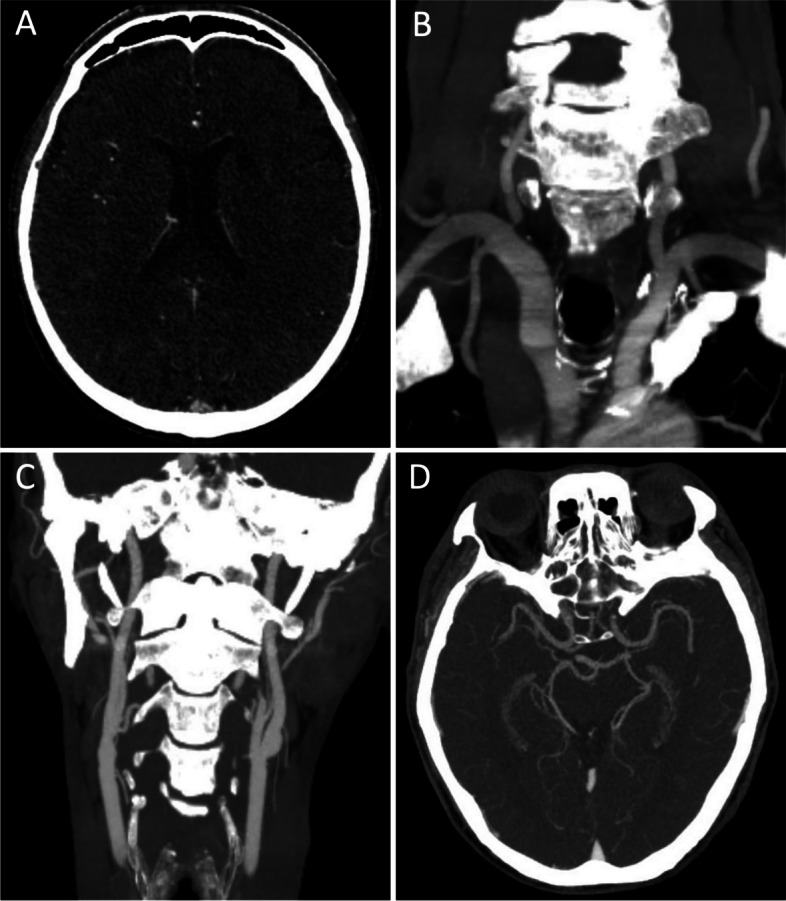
Computed tomography angiogram brain. A Chronic lacunar infarct in left striatocapsular region. B Focal mixed plaque at origin of right vertebral artery causing severe stenosis with preserved distal opacification. C No large vessel occlusion of major cervical vessels. D No major artery occlusion or stenosis in anterior and posterior circulations
